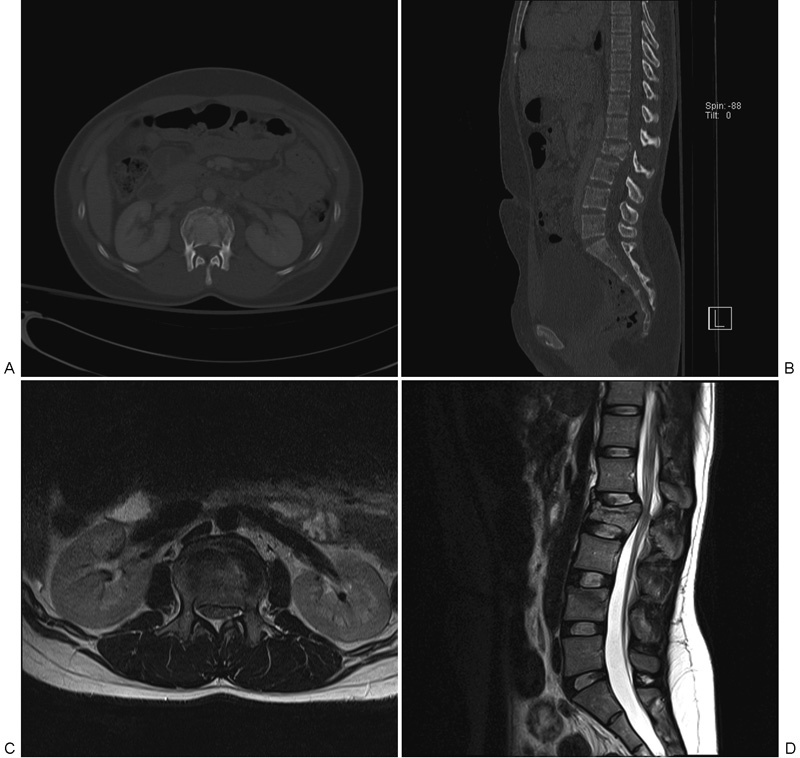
Preoperative assessment of lumbar spine. Burst fracture is noted at L2 vertebra. (A) Axial computed tomography (CT). (B) Sagittal CT. (C) Axial T2-weighted magnetic resonance imaging (MRI). (D) Sagittal T2-weighted MRI.
